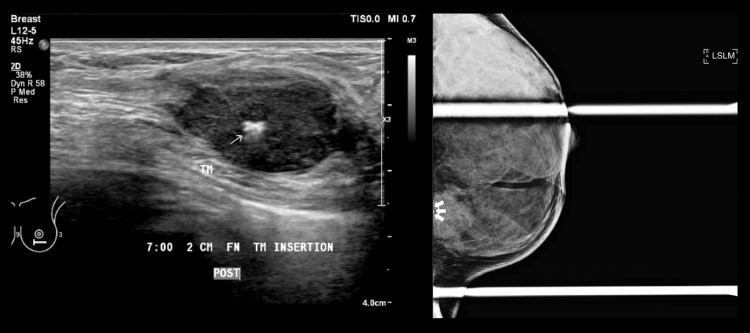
Tumour marker insertion was done under ultrasound guidance. The placement of the marker was confirmed using the SLM view on the mammogram (white arrows). SLM: Straight mediolateral
